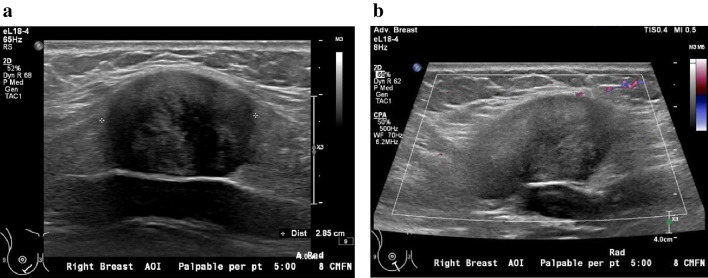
Initial grayscale breast ultrasound (a) demonstrates a well-circumscribed heterogeneously hypoechoic soft tissue mass at the thoracoabdominal wall, measuring 3.8 × 2.0 × 2.9 cm. On color Doppler ultrasound (b), this mass was avascular. The lesion is deep to and separate from the breast parenchyma
